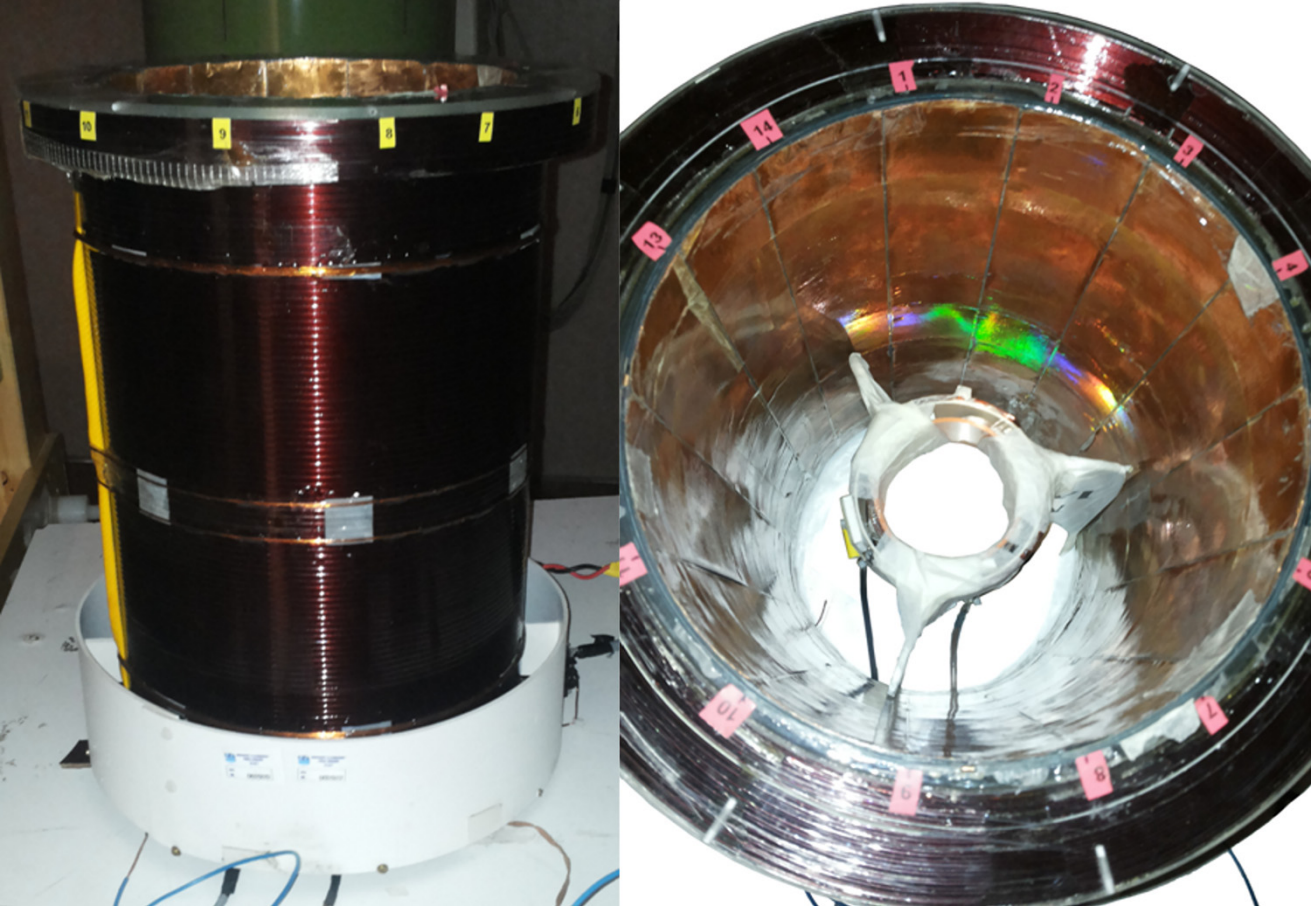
The VLF-MRI coil system. The main compensated solenoid with G_z_ gradient coils (left) and the RF coils placed inside the magnet bore (right).
